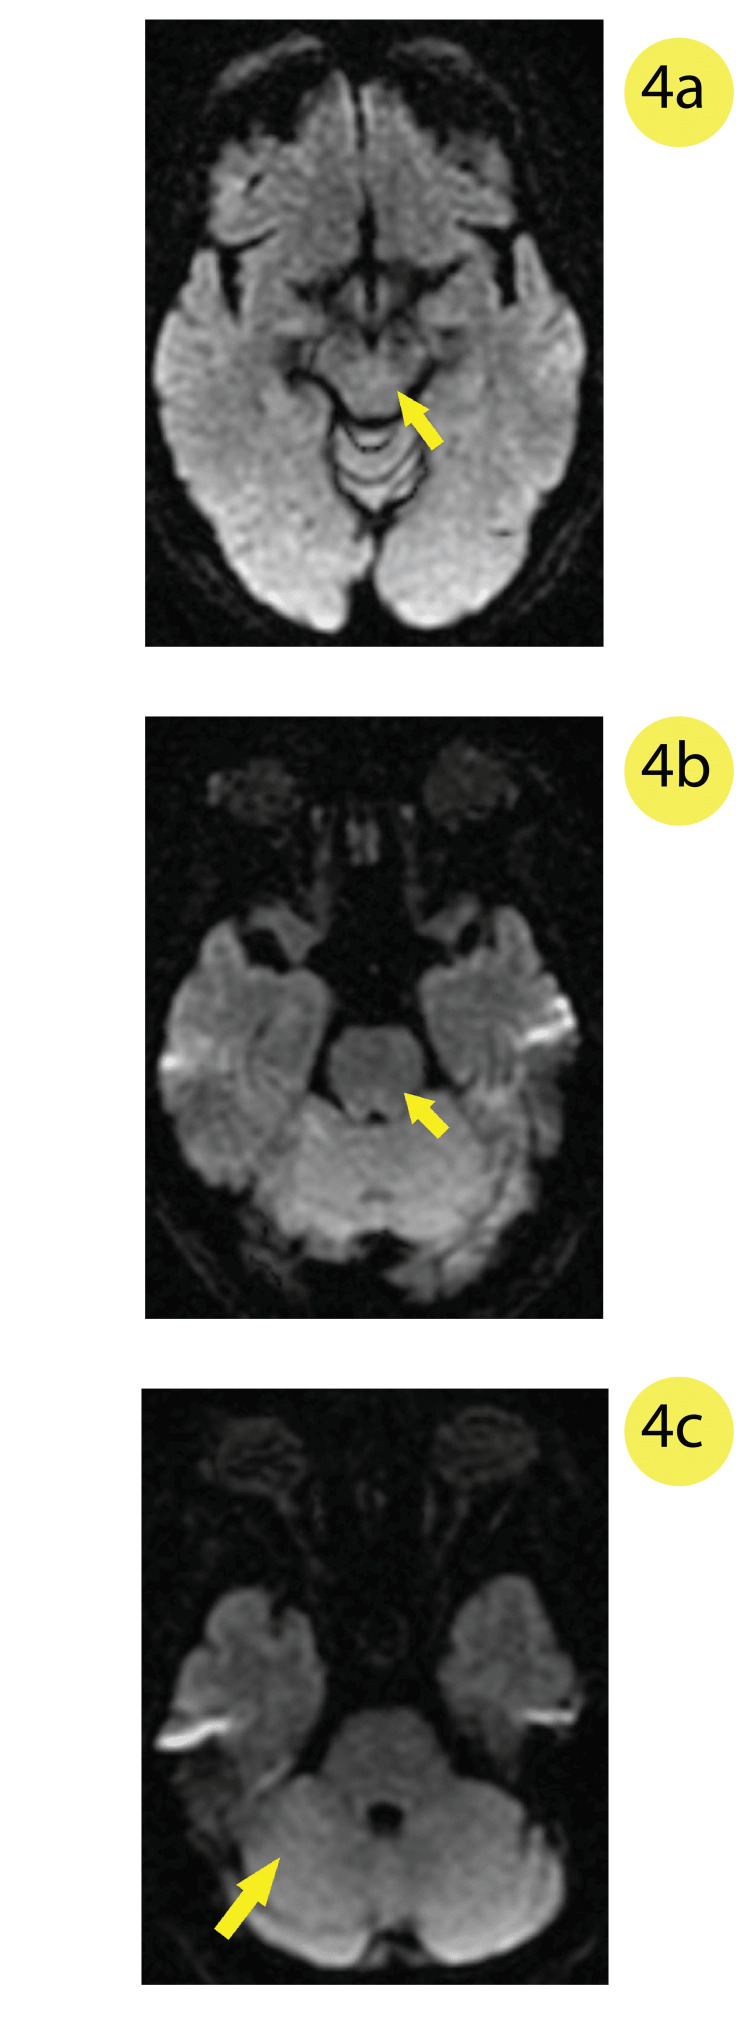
Diffusion-weighted image (DWI) MRI showing no diffusion restriction in the a) central midbrain, b) central-posterior pons, or c) the right superior cerebellum
